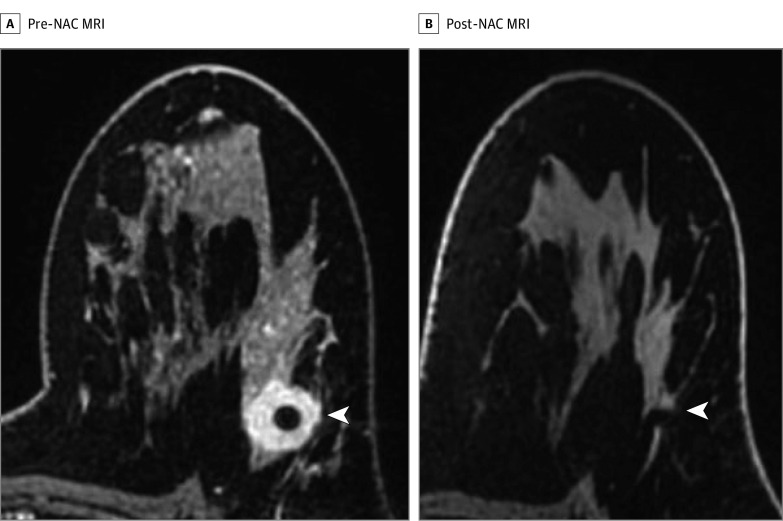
Magnetic Resonance Imaging (MRI) Before and After Neoadjuvant Chemotherapy (NAC) Demonstrating an Imaging Complete Response After NAC A, Axial fat-saturated T1-weighted postcontrast imaging of a biopsy-proven left breast *ERBB2* (formerly *HER2* or *HER2*/neu)–positive invasive ductal carcinoma (arrowhead). B, Axial fat-saturated T1-weighted postcontrast imaging of the same patient demonstrating an imaging complete response defined as no residual tumor enhancement in the treated tumor bed as indicated by the accurately positioned biopsy marker (arrowhead) and anatomic landmarks.
